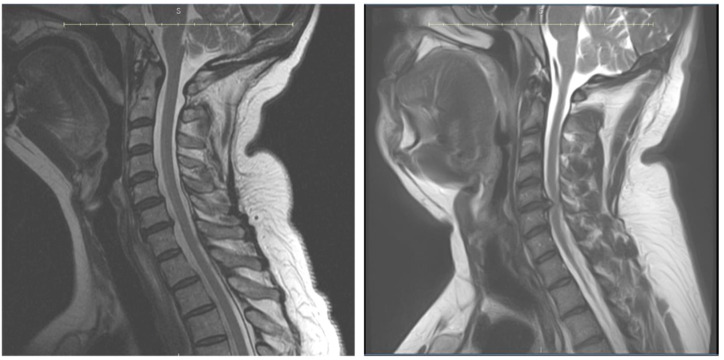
T2-weighted MRI demonstrating a patent cervical canal (left) and single-level stenosis from a large C5/6-disc herniation (right).
